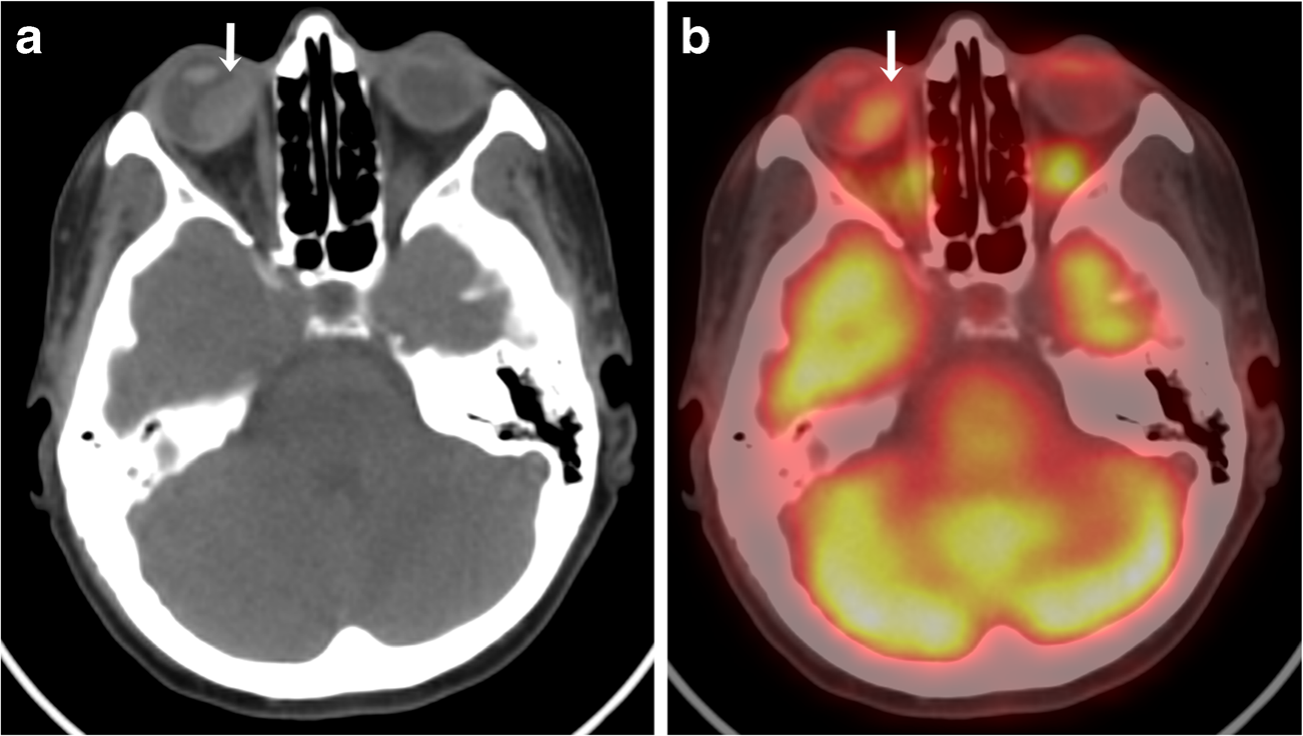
A 62-year-old male with choroidal melanoma. a Axial CT image shows a hyperdense mass in the posterior segment of the right globe (arrow). b The lesion shows moderate FDG uptake on the corresponding axial fused FDG-PET/CT image (arrow)
